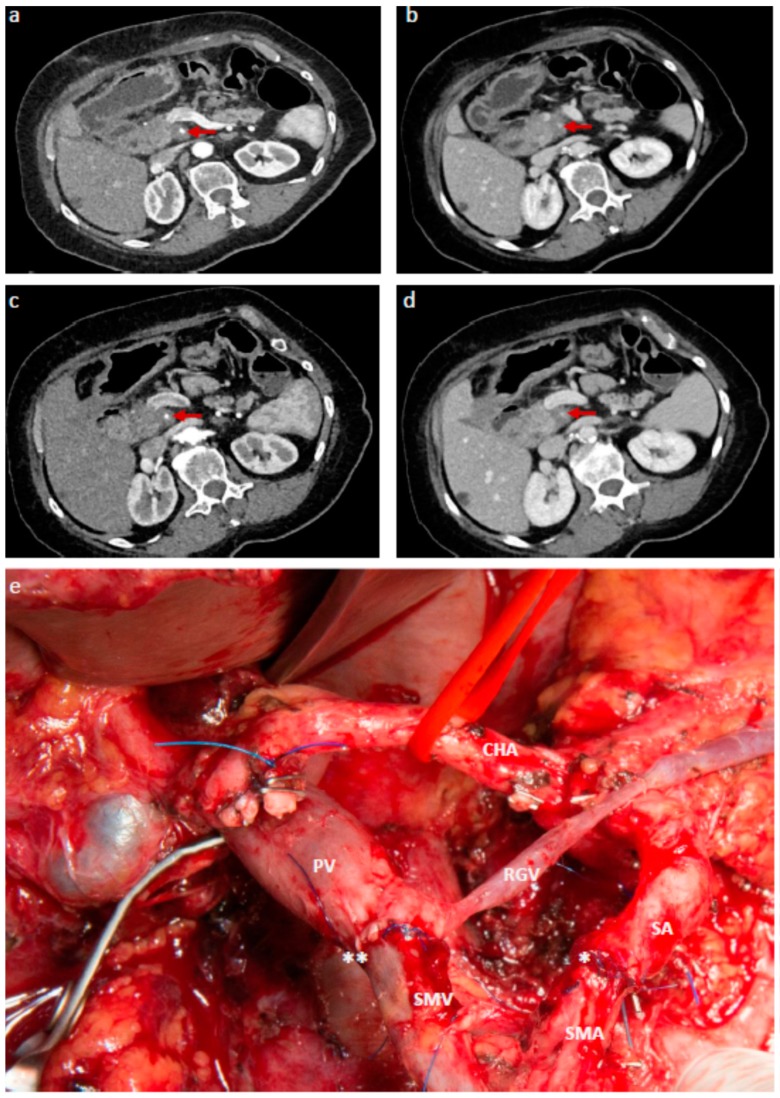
LAPC with stable disease after NAT receiving extended tumor resection with SMA resection: a-d: Transverse multidetector contrast enhanced CT images taken before initiation of NAT (a, arterial phase; b, venous phase) and after completion of six cycles of Gem-based NAT (c, arterial phase; d, venous phase). Unresectable PDAC in the pancreatic body with 360° encasement of the superior mesenteric artery (SMA, red arrow) and occlusion of the superior mesenteric vein (SMV). Stable disease on CT after 5 months (c and d). (e): Operative site after extended tumor resection with the artery-first approach, total pancreatectomy, splenectomy, subtotal gastrectomy, right hemicolectomy, resection of the portal vein (PV) and SMV with direct end-to-end anastomosis (). SMA resection was performed with transposition of the splenic artery (SA), indicated with a single asterisk. Final pathology revealed ypT4, ypN2 (4/54), V1, L1, R1 with no evidence of disease 19 months after surgery.
